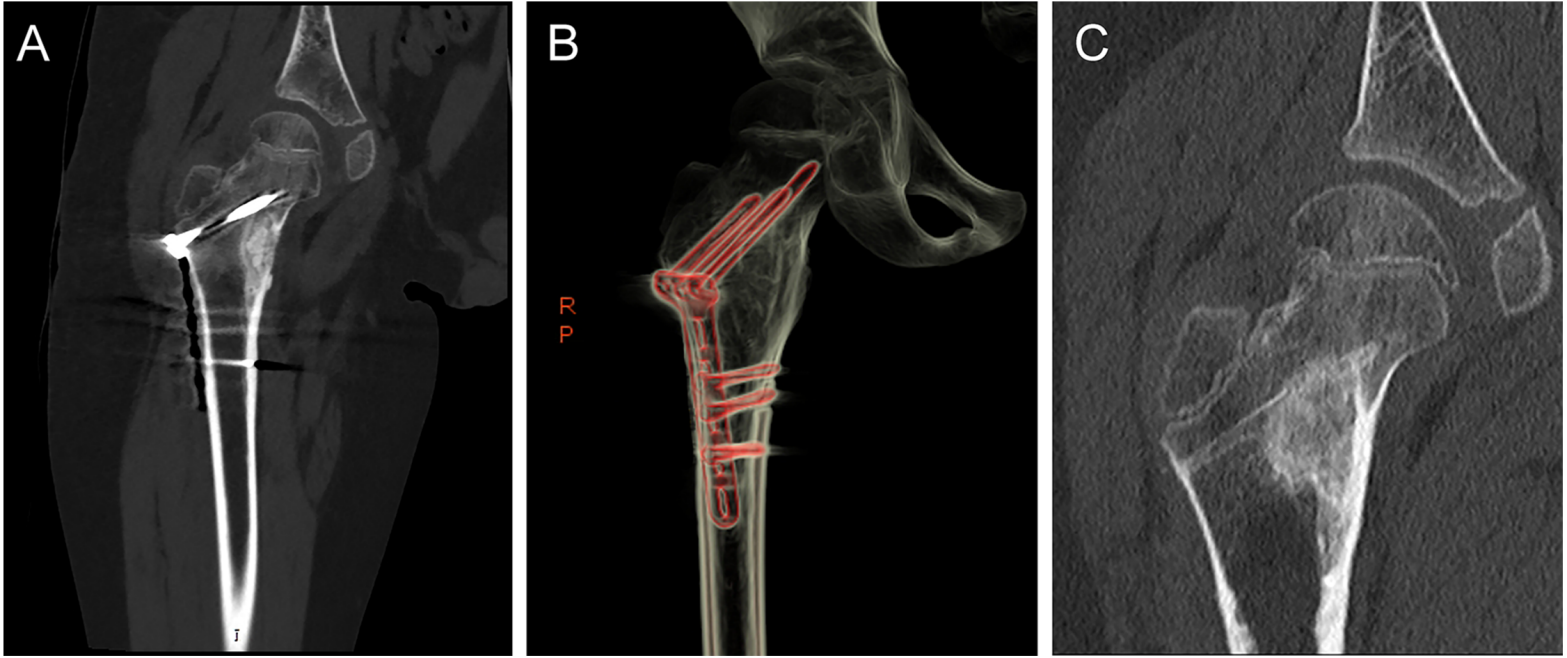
A 9-year-old boy with an osteoid osteoma of the right proximal femur who underwent surgical focal resection followed by bone grafting and internal fixation with plate screws. (A) Post-operative coronal CT image showed that the residual cavity of the lesion area was filled with dense allogeneic bone, and metal internal fixation screws and plates were visible. (B) Three-dimensional reconstruction image after screw and plate internal fixation. (C) One year after surgery, the CT scan was repeated, and the internal fixation device was removed, and the density and size of the lesion remained the same.
